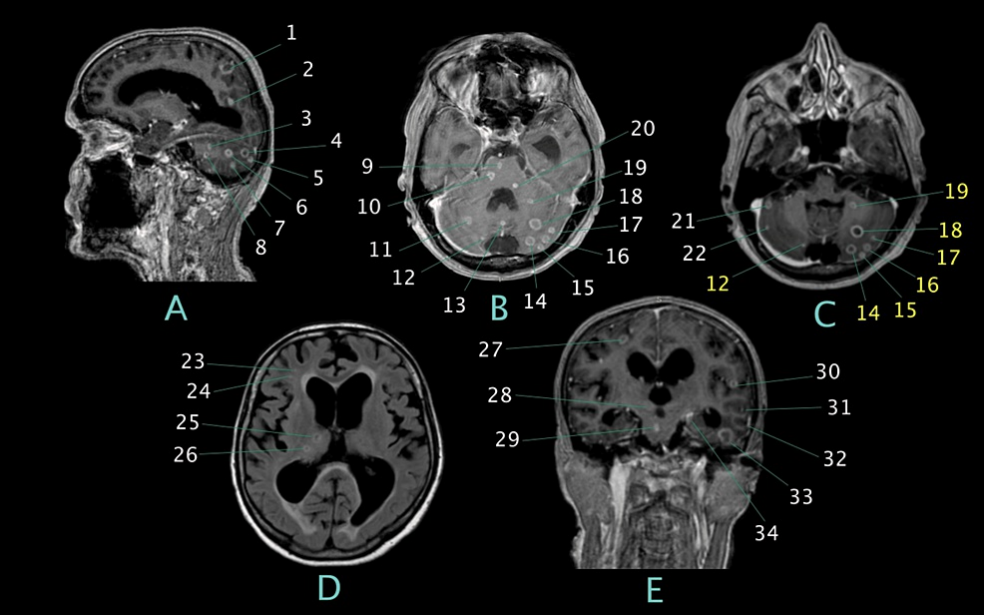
Contrast-enhanced T1-weighted MRI showing 34 tuberculomas. Sagittal (A), axial (B-D), and coronal (E) planes. Due to their number, the lesions are indicated by thin lines. Numbers in yellow indicate duplicate lesions. Unlabeled abnormalities include ventricular dilation due to communicating hydrocephalus, periventricular leukoencephalopathy, and cortical atrophy.
